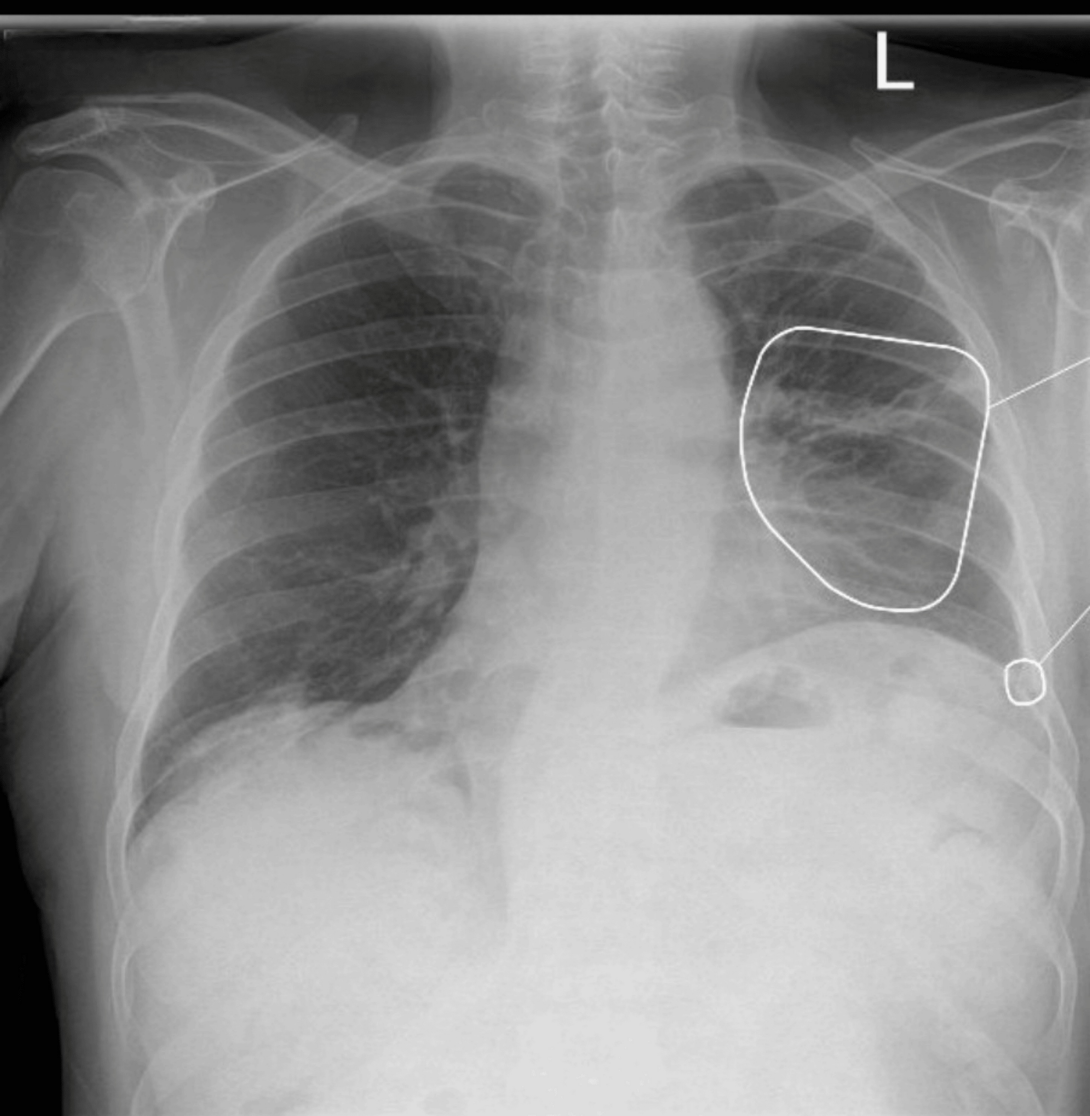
Follow up chest radiograph
